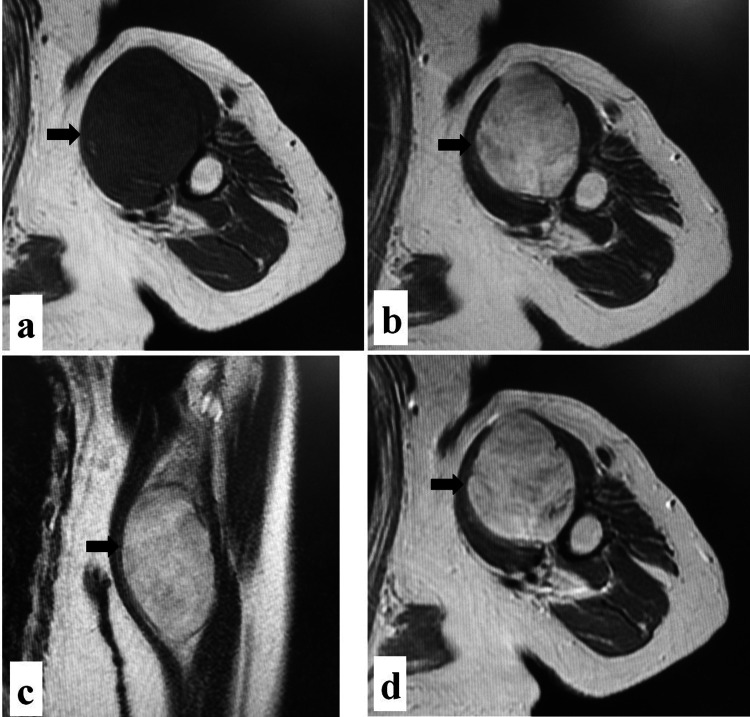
Magnetic resonance images of the left upper arm. (a) Axial T1-weighted, (b) axial T2-weighted, (c) coronal T2-weighted, and (d) axial T1-weighted contrast-enhanced images showing a tumor with iso signal intensity on T1-weighted, mild hyperintensity on T2-weighted, and whole-tumor enhancement on contrast-enhanced images (arrows).
